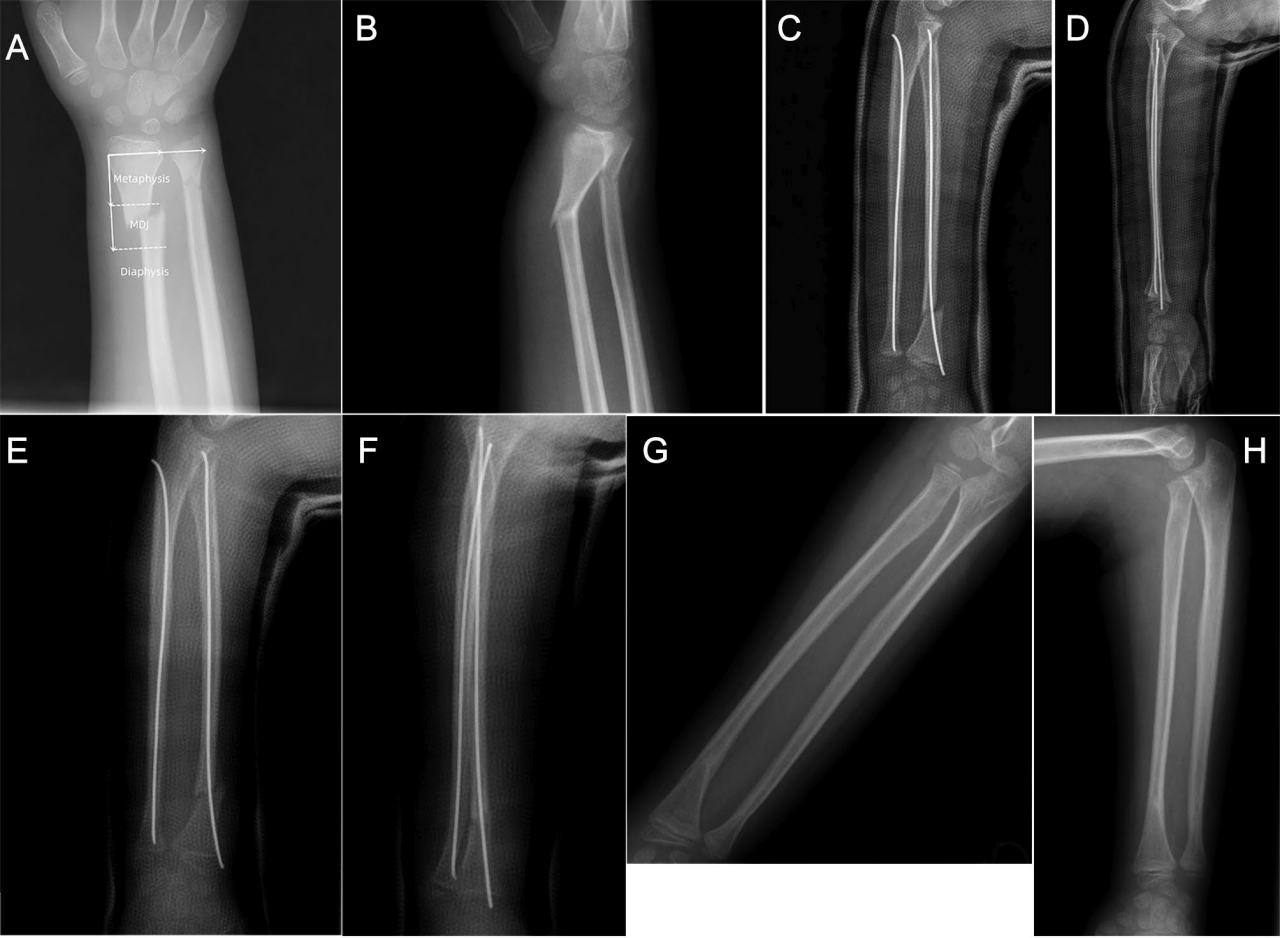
(A) Definition of an MDJ fracture of the distal radius. A 9-year-old boy presented with a right distal radius and ulna. (A, B): Radial fractures are observed in the MDJ. (C, D): Closed reduction and ESIN are performed. (E, F): Radiographic examination performed 1 month after the surgery reveals radiographic union. (G, H): The elastic nail was removed 4 months after the surgery. Final follow-up findings show fracture healing and no deformities. MDJ: metaphyseal-diaphyseal junction; ESIN: elastic stable intramedullary nailing
